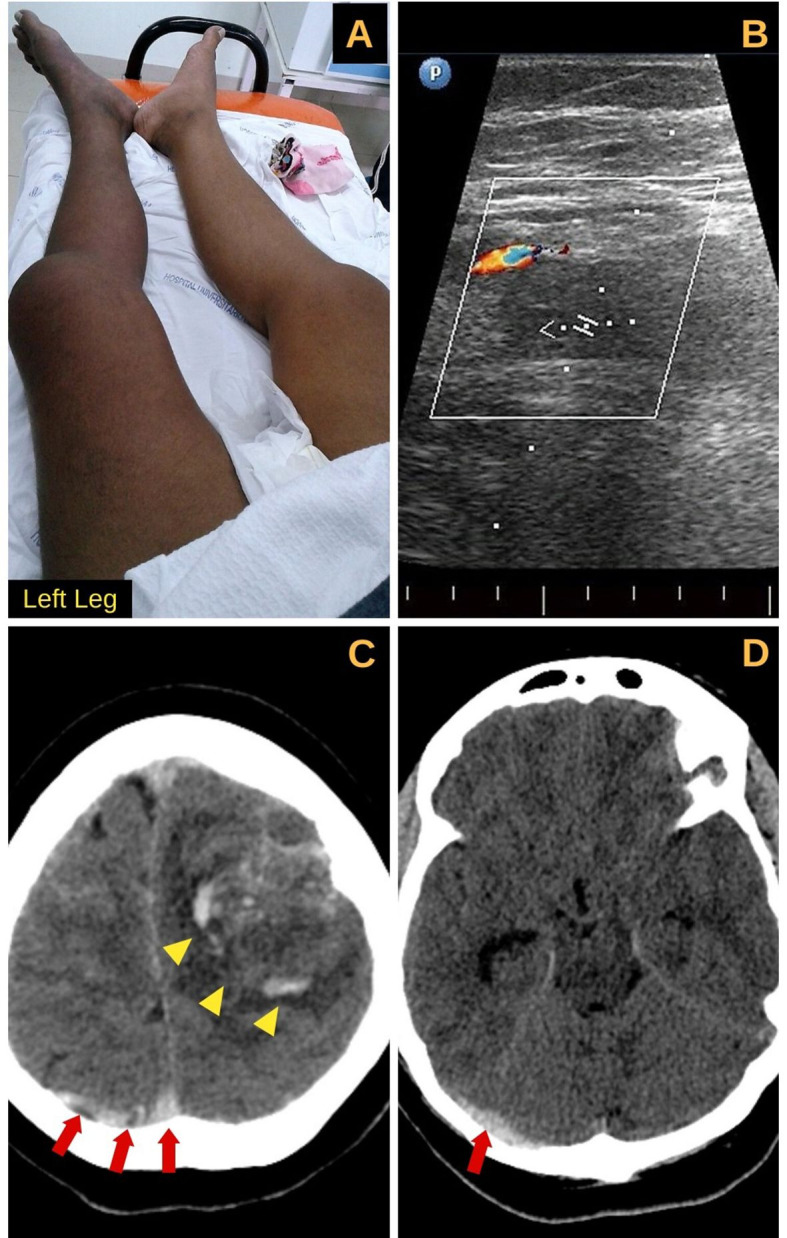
Images from Case: Massive left leg edema with cyanosis (A). Venous ultrasound with Doppler of the common left iliac vein showing no venous flow and absence of compression; signs compatible with deep vein thrombosis (B). Non-contrast enhanced computed tomography scan showing signs of cerebral venous thrombosis. The images show dense clot signs in the sagittal and right transverse sinuses (red arrows) (C, D) and left temporoparietal hemorrhage with surrounding edema (yellow arrowheads) (C).
